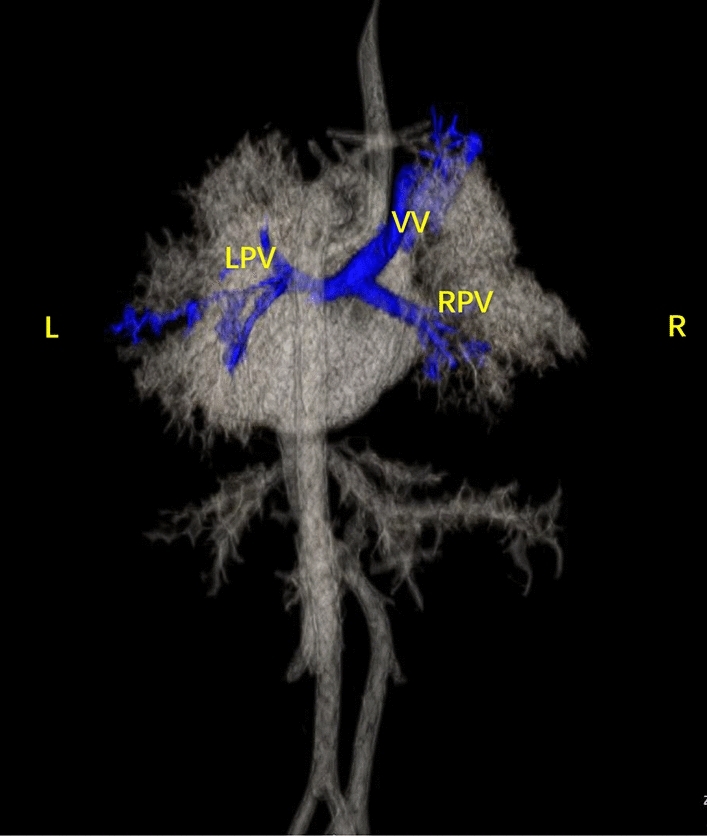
Postpartum CTA findings. The left and right PVs converged to form a PV trunk and returned to the right superior vena cava via the vertical vein.
